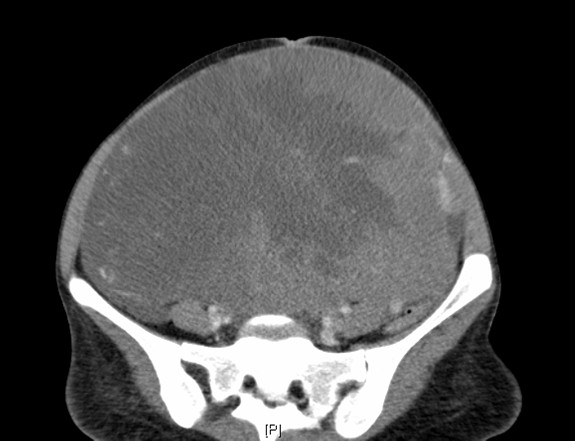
CT scan of the abdomen on admission. This scan demonstrates a large necrotic, partially cystic tumour occupying most of the abdomen. The CT scan also demonstrated marked paraaortic lymphadenopathy but no visceral or CNS metastases.
